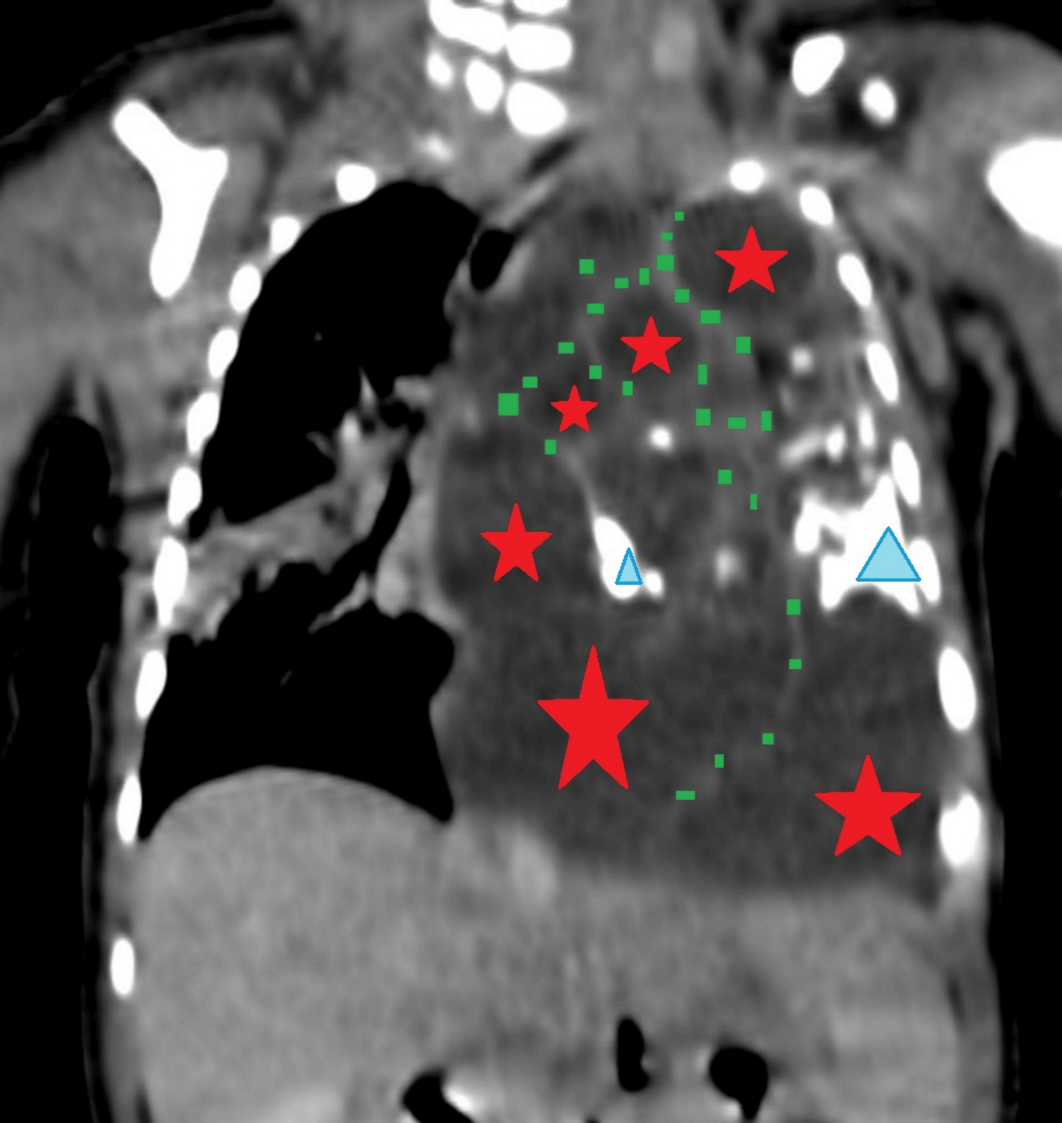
Injected thoracic CT scan objectifying the teratoma (coronal section) Injected thoracic CT scan at arterial phase in the mediastinal window demonstrating a voluminous mass with three components: cystic (red marks), tissue (green mark), and calcified (blue marks), associated with deviation of mediastinal structures toward the contralateral side.
